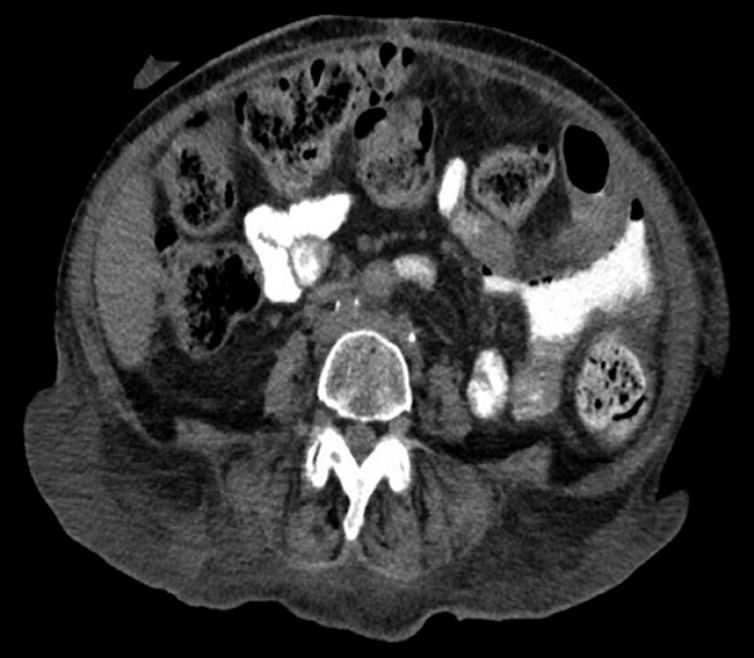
Computed tomography image of the abdomen showing extraluminal contrast extravasation within the left upper quadrant, raising concern of an anastomotic leak.
